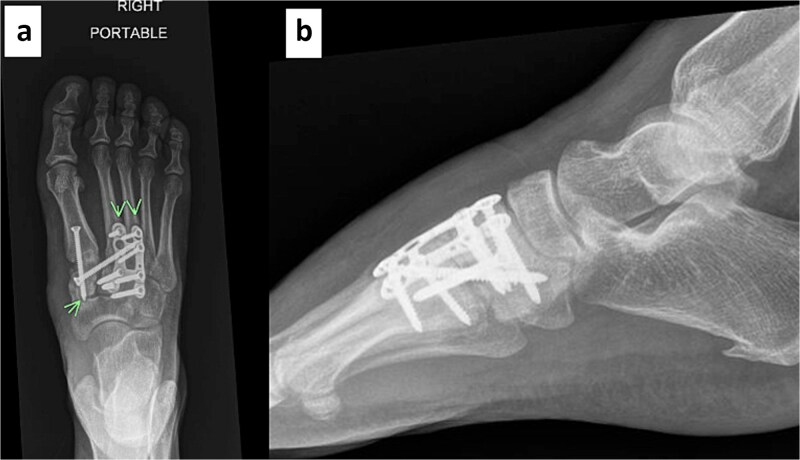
(a–b) Radiographs at 6 months demonstrate maintained reduction of the first through third tarsometatarsal joints without hardware failure or recurrent displacement.
